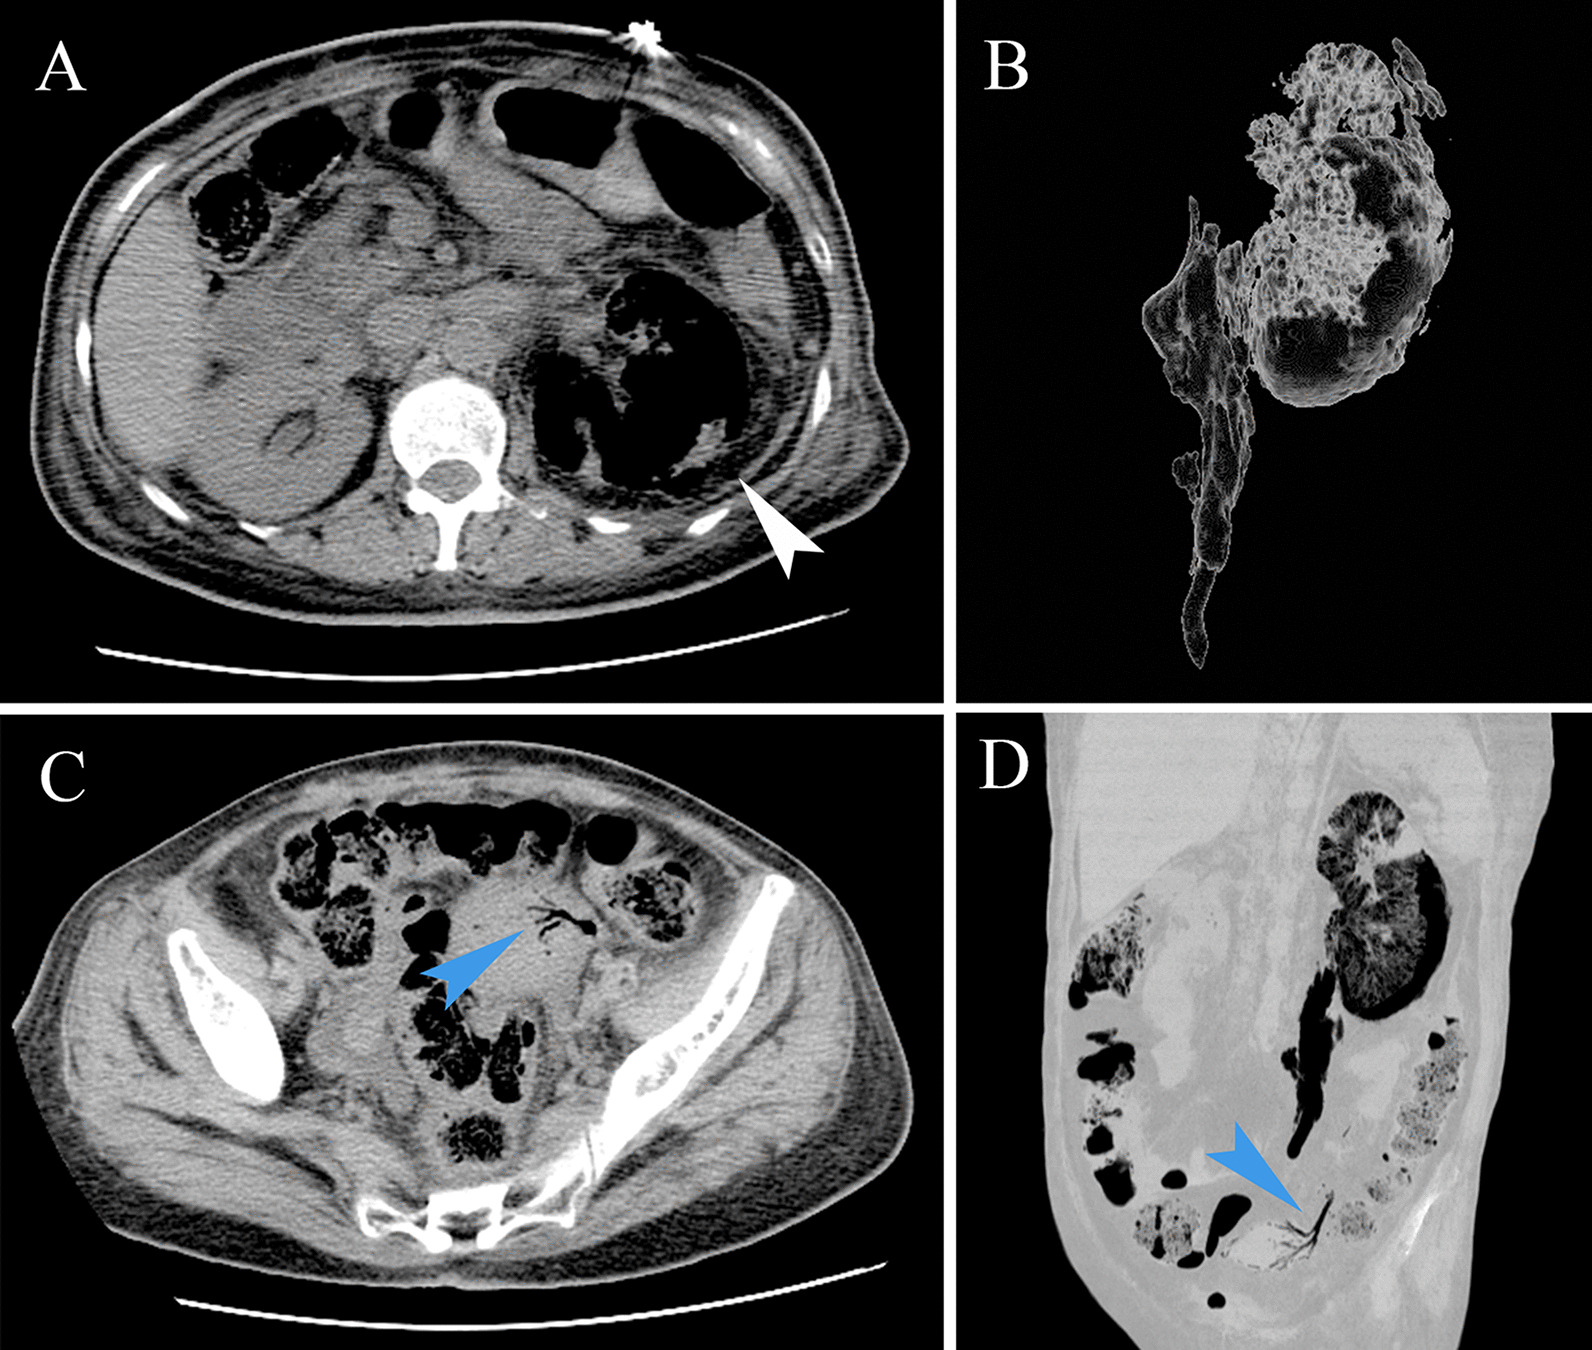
Images in 53-year-old female with emphysematous pyelonephritis (EPN) of the left kidney. A, B Axial and volume-rendered abdominal CT shows the presence of gas in the parenchyma, para-renal space and left ureteral upper and middle part (white arrow). C, D Axial and multiplanar reconstruction in minimum-intensity projection CT shows air bubbles in the uterine vein (blue arrow)
